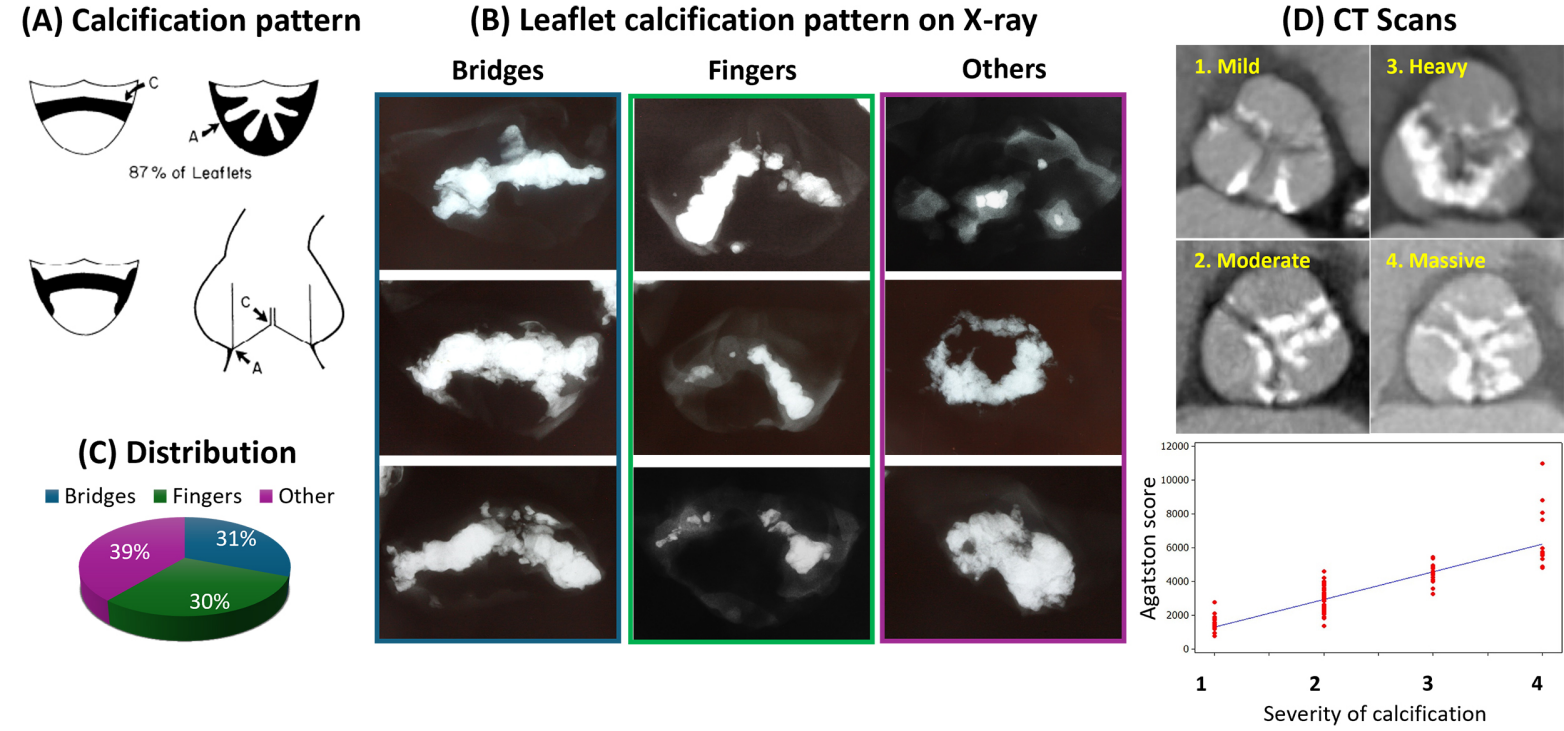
Calcification Patterns Seen in Radiography and Computed
